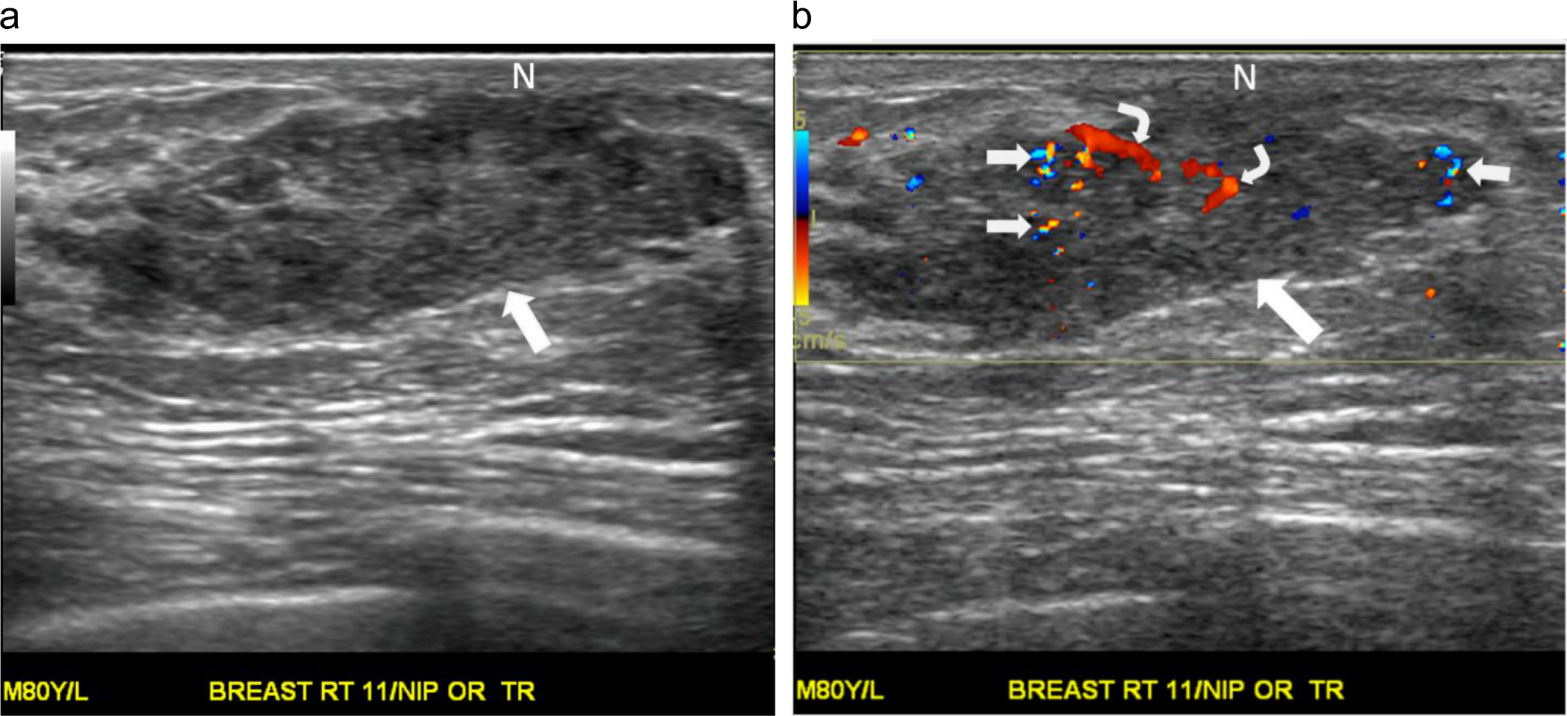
A palpable mass in the right breast of an 80-year-old man. (a) Transverse ultrasound shows an oval shape, a noncircumscribed margin, and a heterogeneously hypoechoic, solid nodule (arrow). The nodule has a parallel orientation and a mildly eccentric relationship to the nipple (N). (b) Color Doppler ultrasound shows multiple color pixels (short arrows) and two pedicles of blood supply (curve arrows) in the nodule (long arrow), categorized as a rich color flow signal with predominantly internal vascularity. The postoperative pathological diagnosis is gynecomastia.
